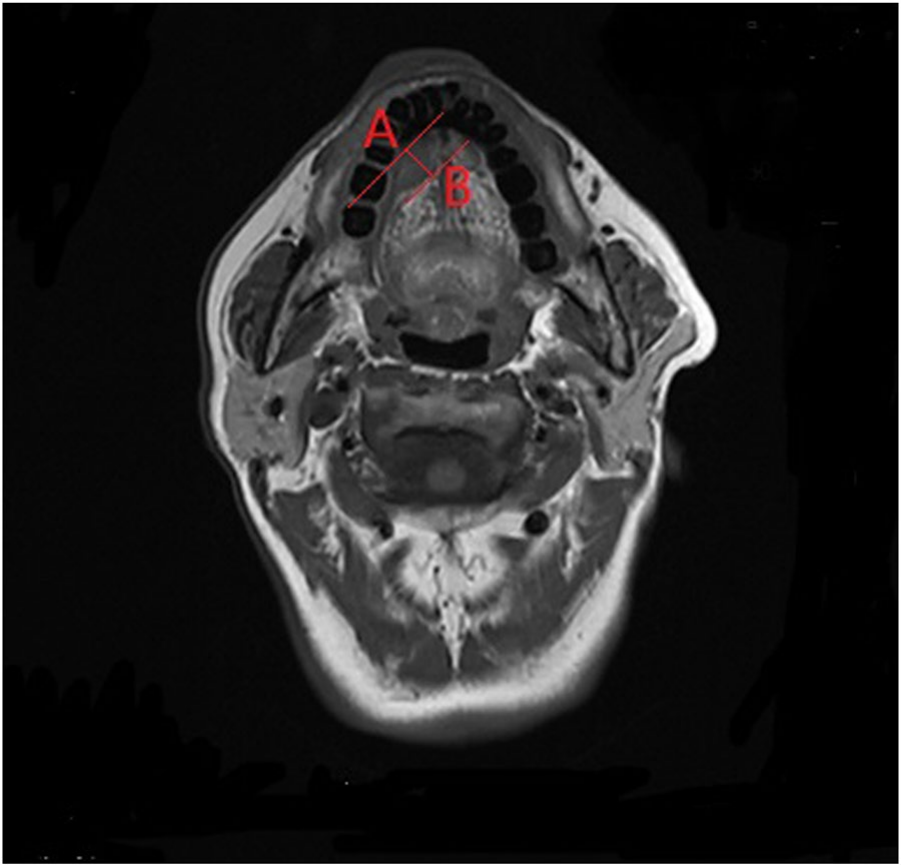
Measurement of the MRI-determined depth of invasion (distance between A and B points) based on the adjacent normal mucosal junction to the deepest infiltration point.
